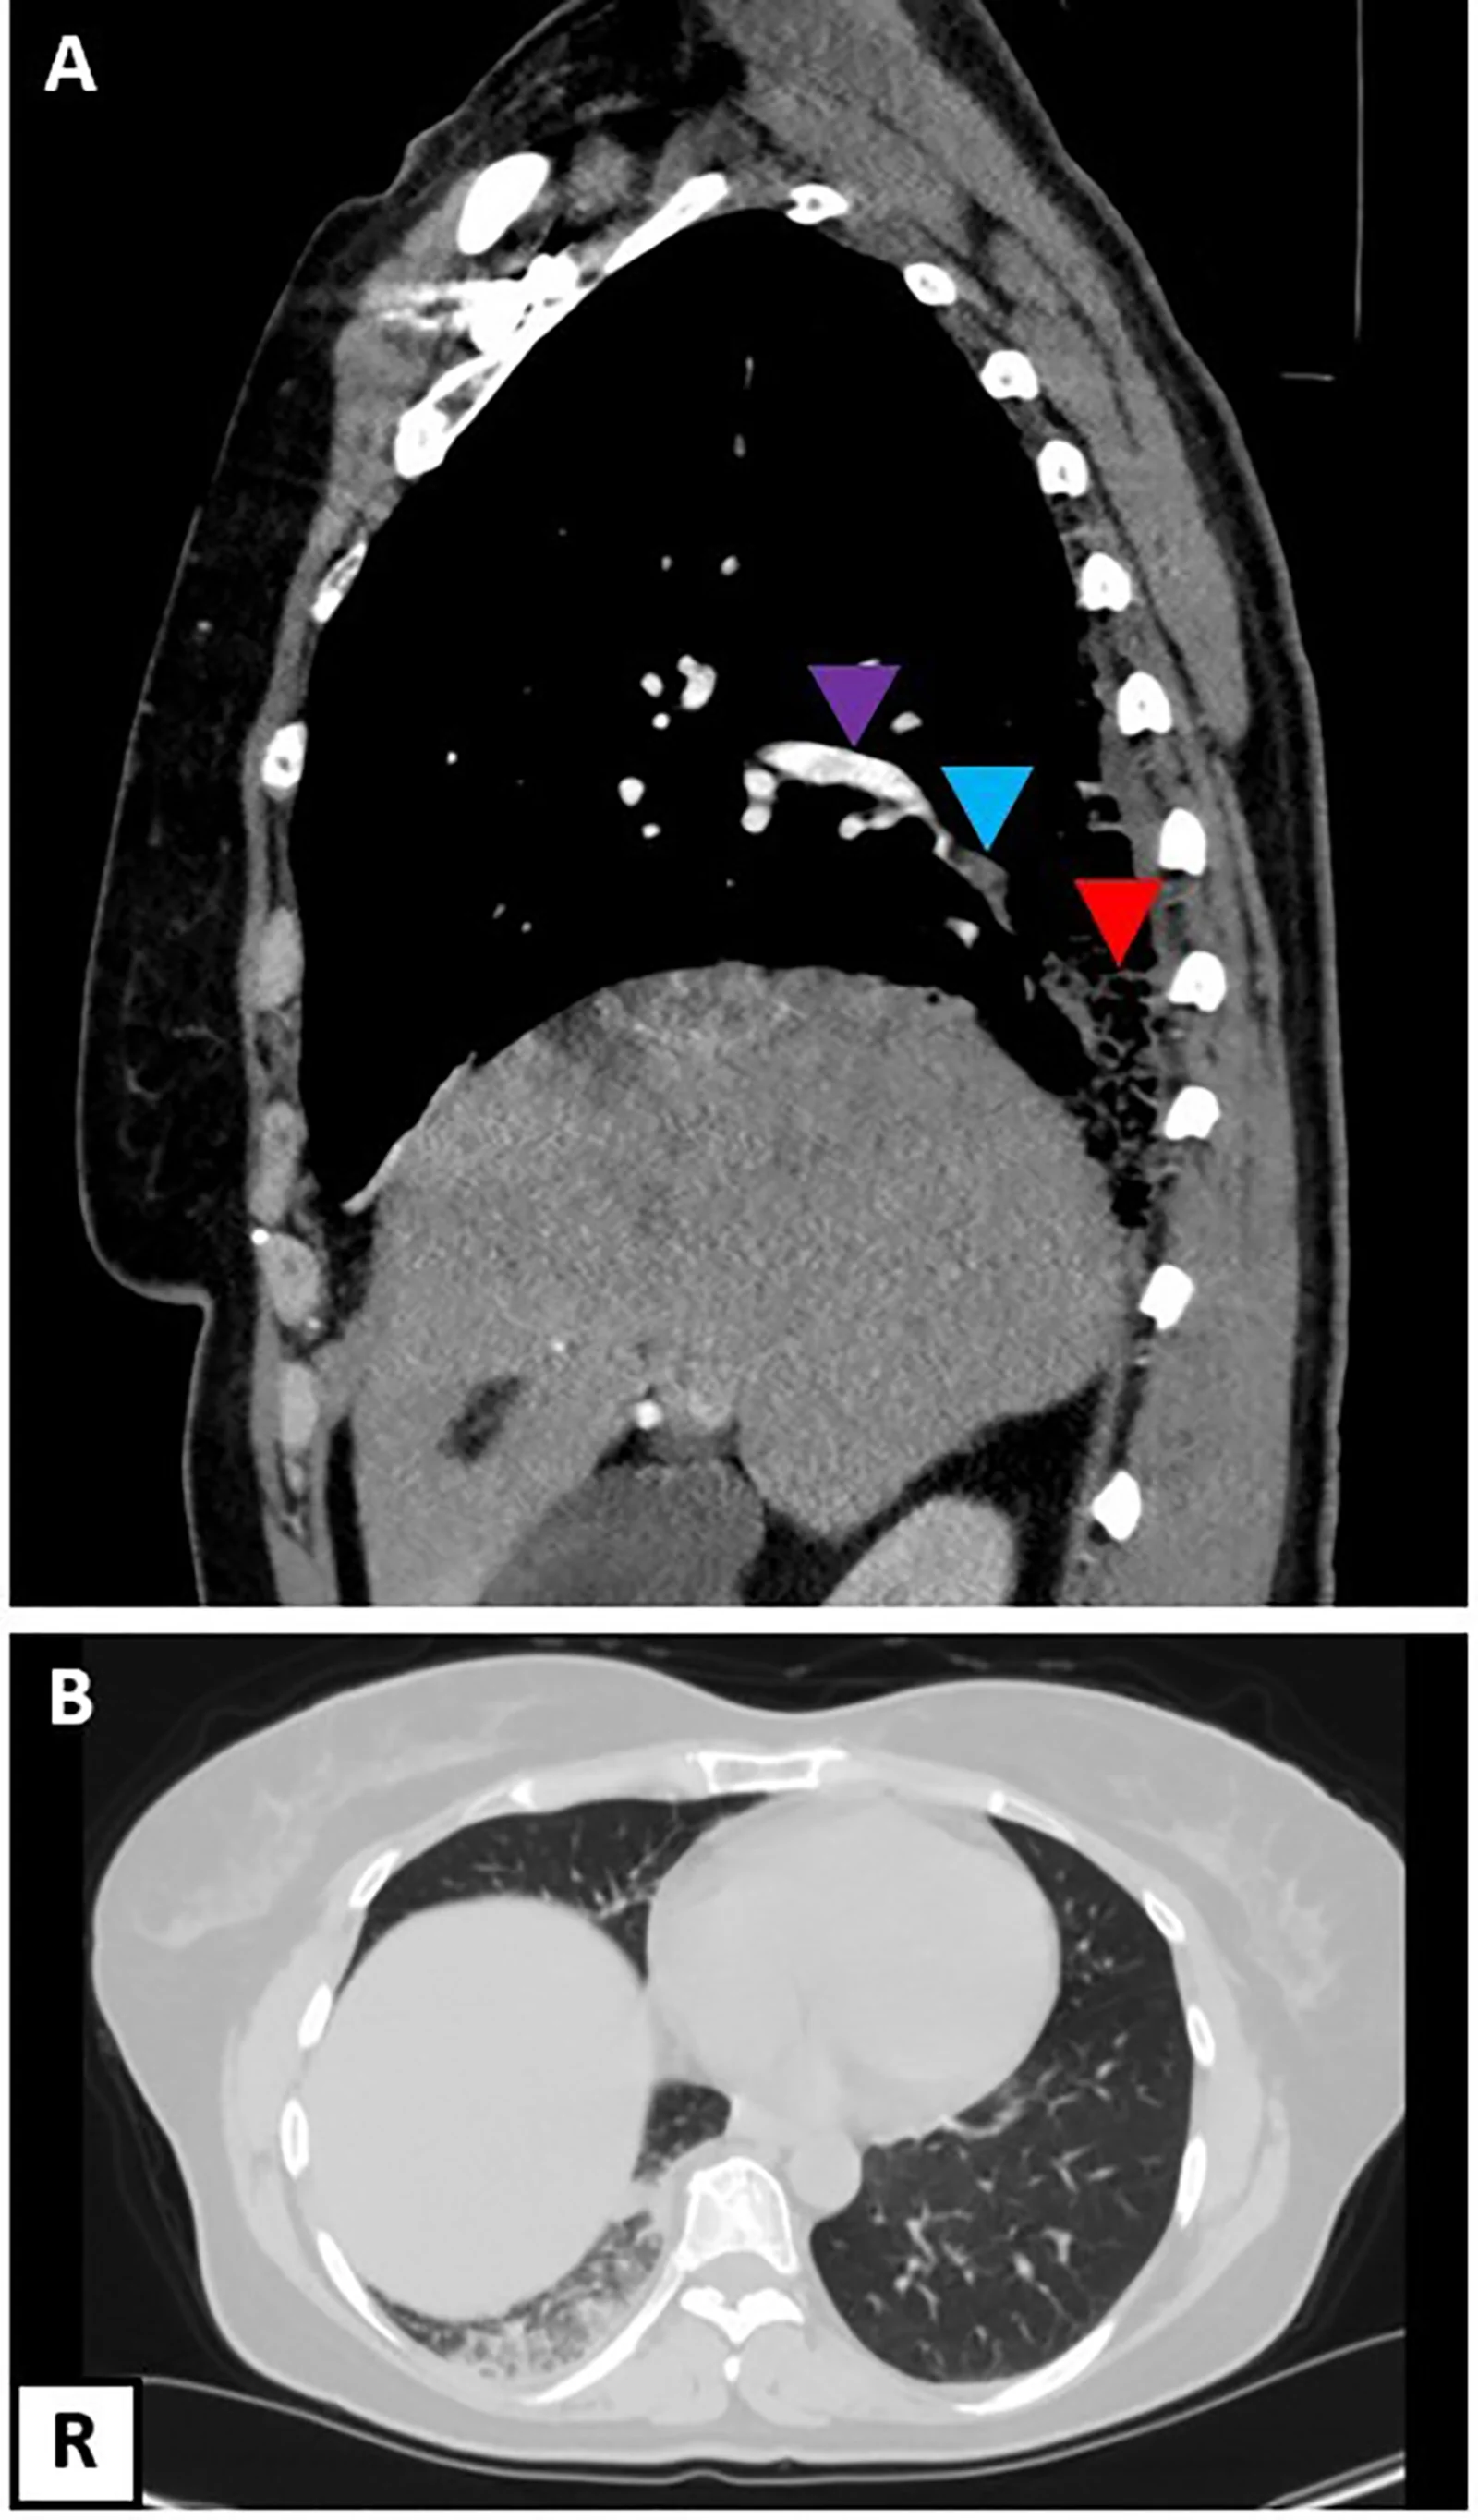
(A) A sagittal view of the right chest showing the obstructing embolus represented as a filling defect (blue head arrow) with proximal dilatation of the right pulmonary artery branch (corresponding to the Palla sign on chest x-ray) marked by the purple head arrow. Additionally, right base collapse is seen (red head arrow). (B) A lung window showing the presence of consolidations at the right lung base, as well as an elevated right diaphragm.
